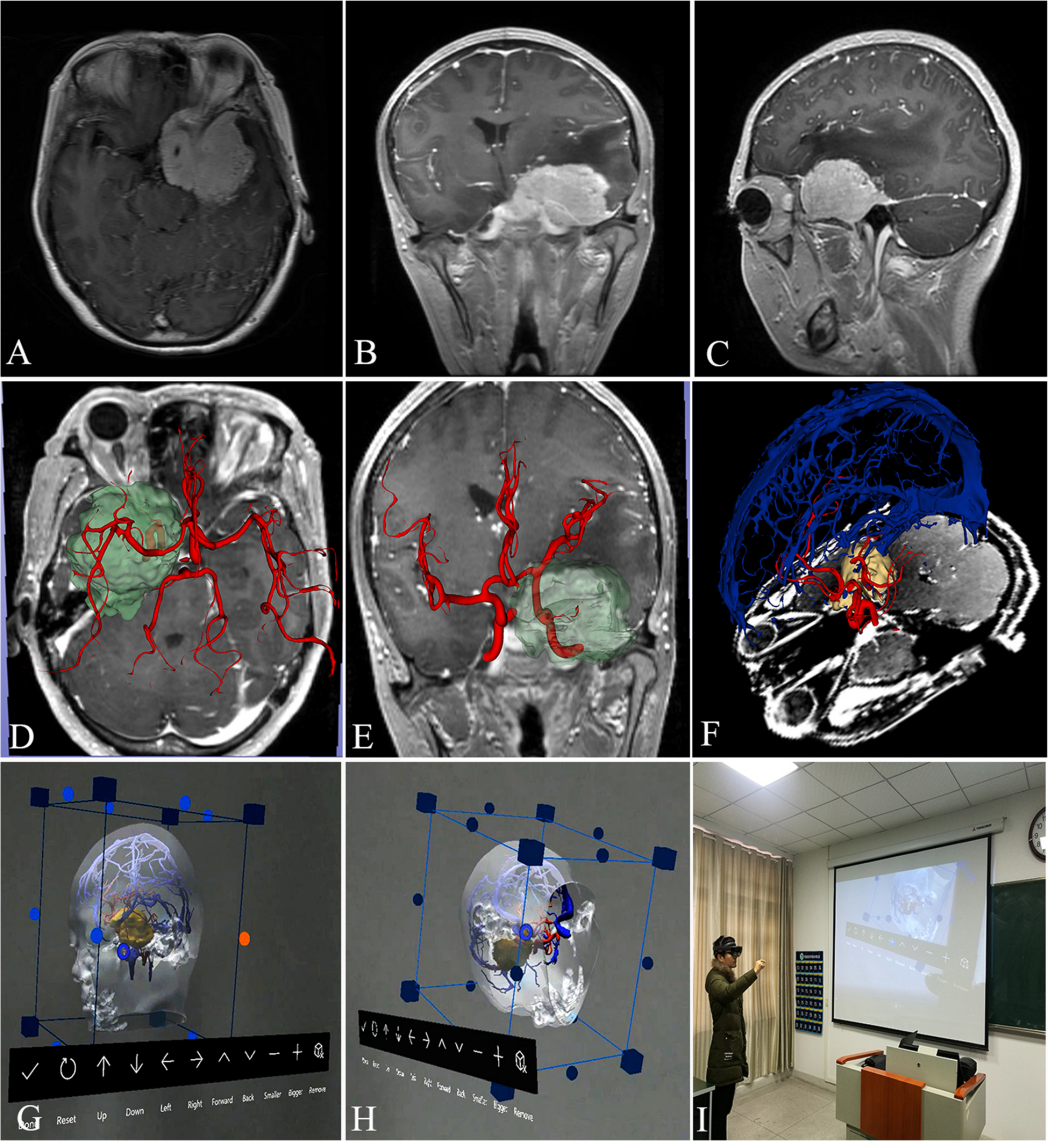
Virtual reconstruction of a left sphenoid ridge meningioma in one patient. a-c MRI results. d-f 3D display of MRI. g, h The images viewed by the Microsoft Hololens glasses. h The image of one student using the Microsoft Hololens glasses
